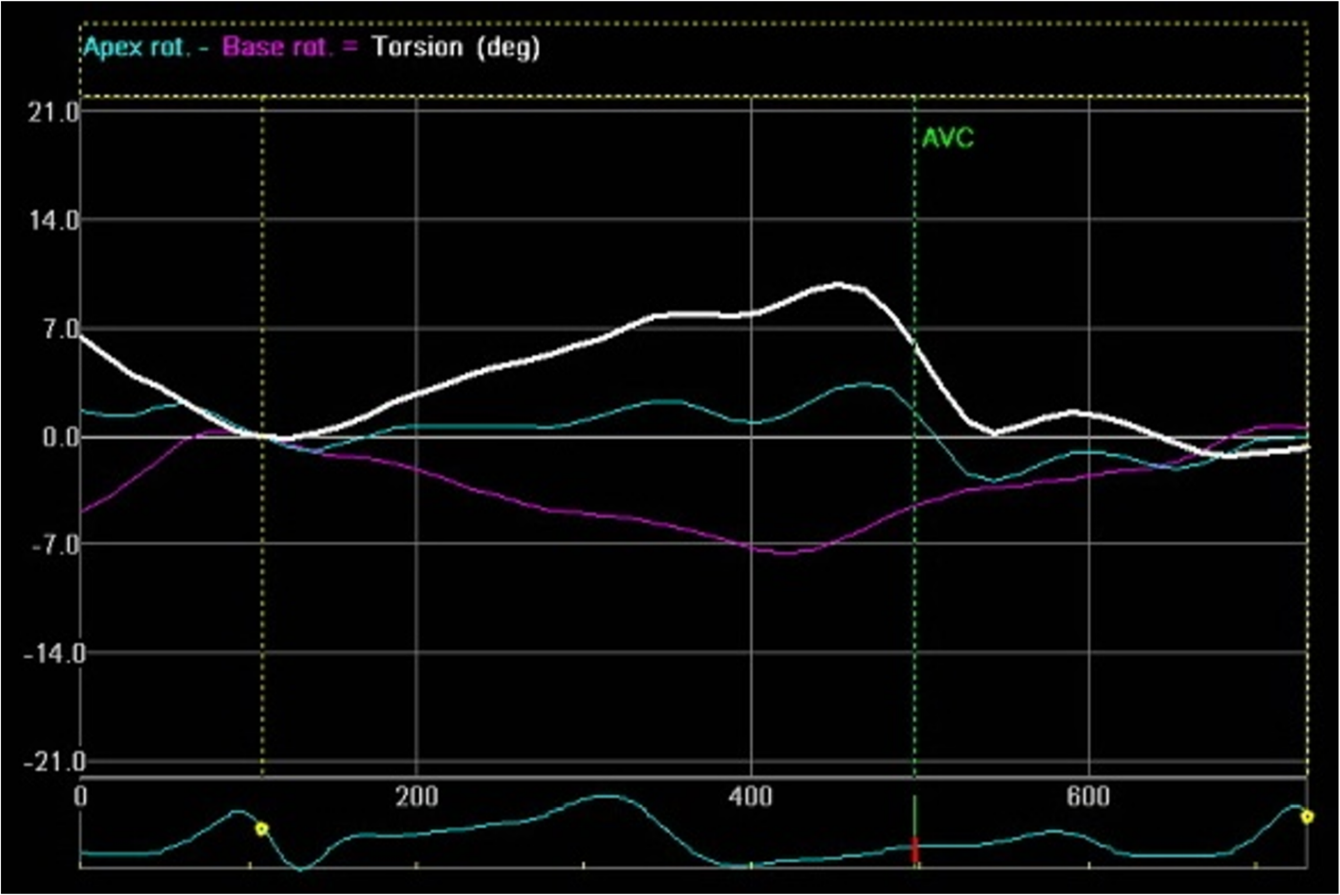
Speckle tracking echocardiography (Echo Pac software) in normal subjects showing net basal rotation (purple color) in counter-clockwise direction and net apical rotation (green color) in clockwise direction. Net torsion is displayed in white color
